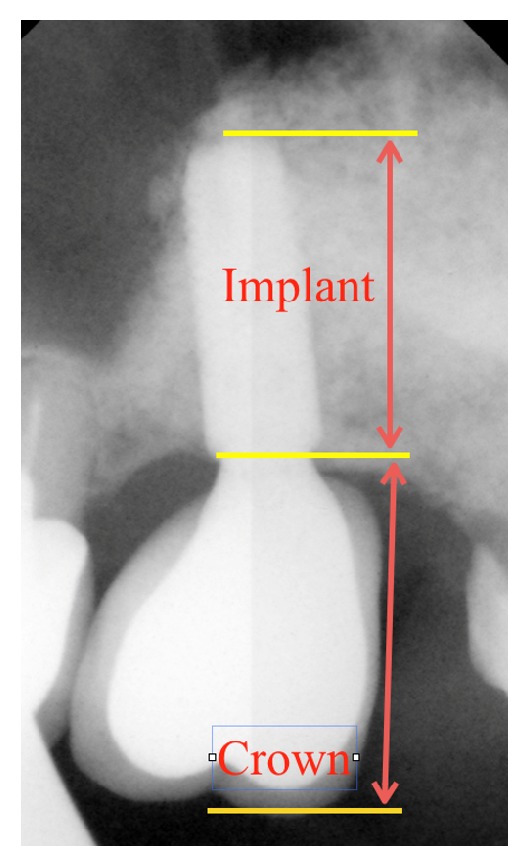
Periapical digital radiograph of a regular implant (Astra Tech implant system OsseoSpeed TX 4.0 S; Ø4 mm, 11 mm long). The *C*/*I* ratio measurement method is presented. Radiological status 36 months after implant placement.
